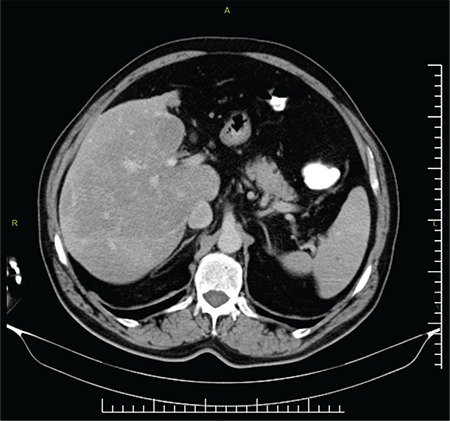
Computed tomography of the abdomen showed nodularity of the surface of the liver, a heterogeneous appearance of the liver parenchyma, and atrophy of the left liver lobe.
